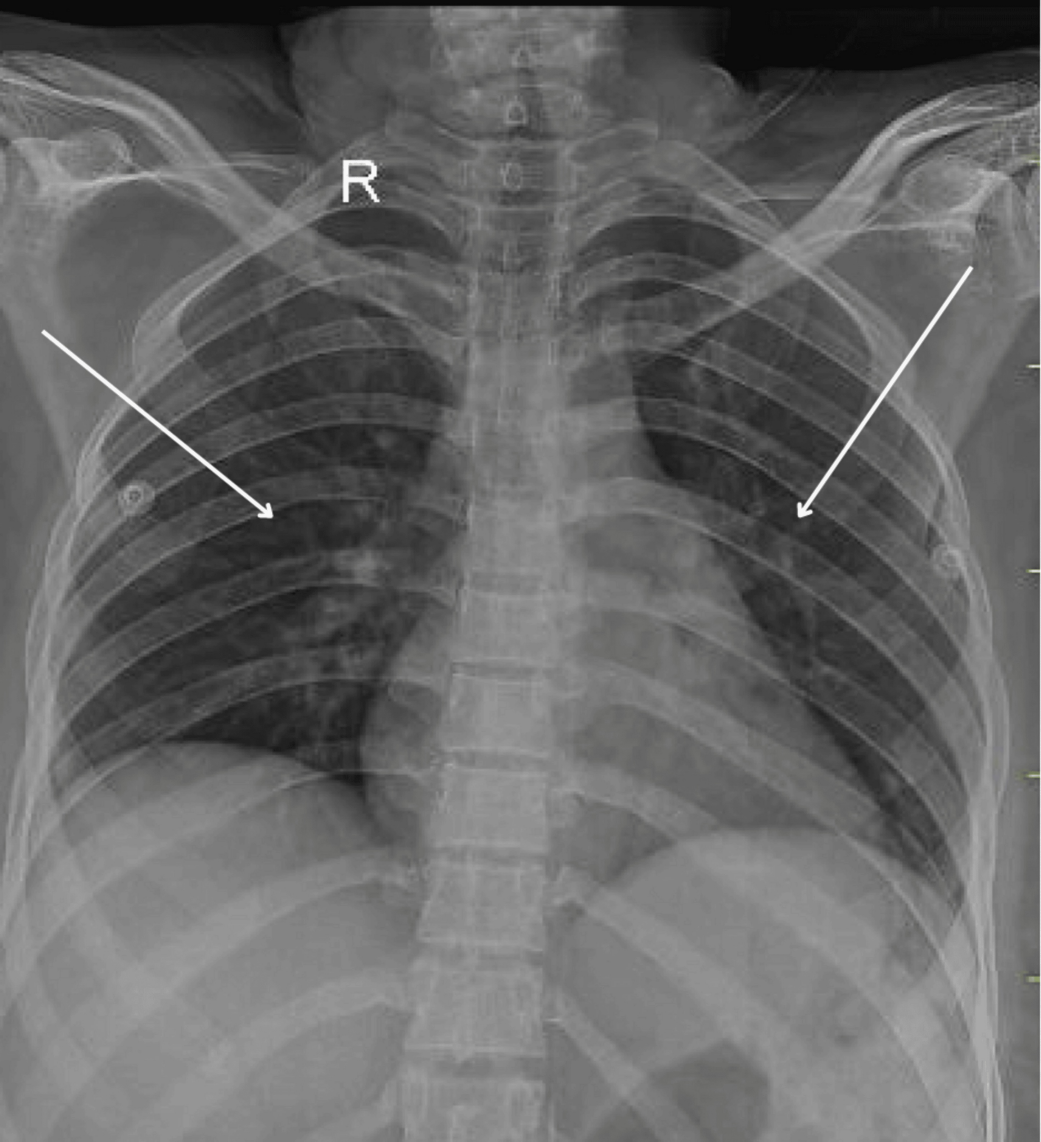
Chest radiograph with clear lung fields indicating the resolved pulmonary edema (white arrows)
